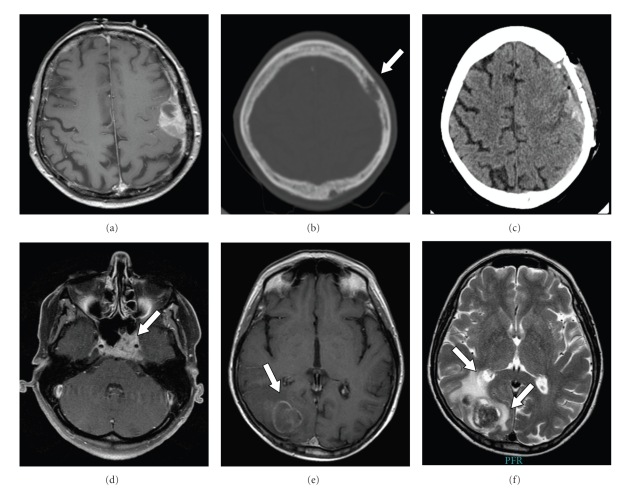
(a)–(c) 77-year-old femal with aphasia resulting from breast cancer dural metastasis, (a) preoperative T1-weighted MRI showing contrast enhancement of a left parietal dural tumor. (b) Preoperative CT scan shows bone erosion (arrow). (c) Postoperative CT scan documents the removal of the dural mass and the infiltrated bone which has been substituted by bone cement cranioplasty. (d) T1-weighted MRI showing contrast-enhancing breast cancer skull base metastasis in the clival region occurring in a 45-year-old female with known breast cancer and diplopia (arrow). (e, f) MRI of a 69-year-old female with right occipital breast cancer brain metastases. (e) T1-weighted MRI shows a ring-like contrast-enhancing lesion (arrow). (f) T2-weighted MRI shows the lesion with significant peritumoral finger-like edema (arrows).
